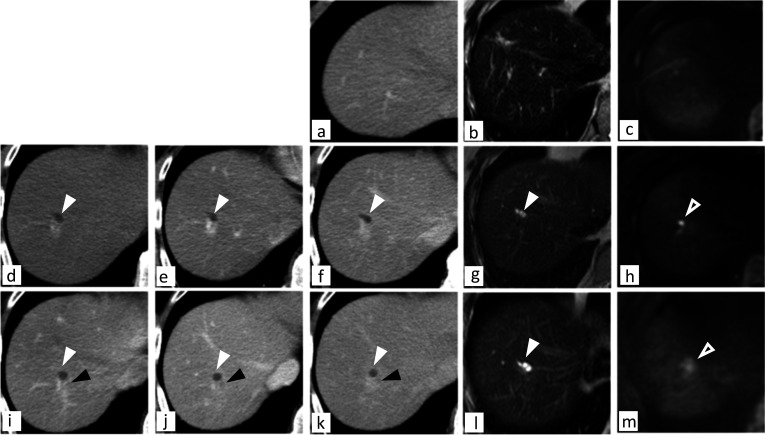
Temporal changes in abdominal CT and MRI findings. (a) Single-phase contrast-enhanced CT; (d, i) hepatic artery phase; (e, j) portal phase; and (f, k) equilibrium phase in dynamic abdominal CT imaging. T2-weighted (b, g, l) and DWI (c, h, m) MRCP images. Absence of abnormalities in the intrahepatic bile ducts during initial examination. (a, b, c) Images showing dilatation of B8 (d, e, f, g) and a small area of high signal intensity observed on DWI (h). Preoperative imaging revealing a tumor with ring enhancement at the root of B8 (i, j, k, l), corresponding to that area, and the high-signal intensity on DWI imaging increasing in size (m)
